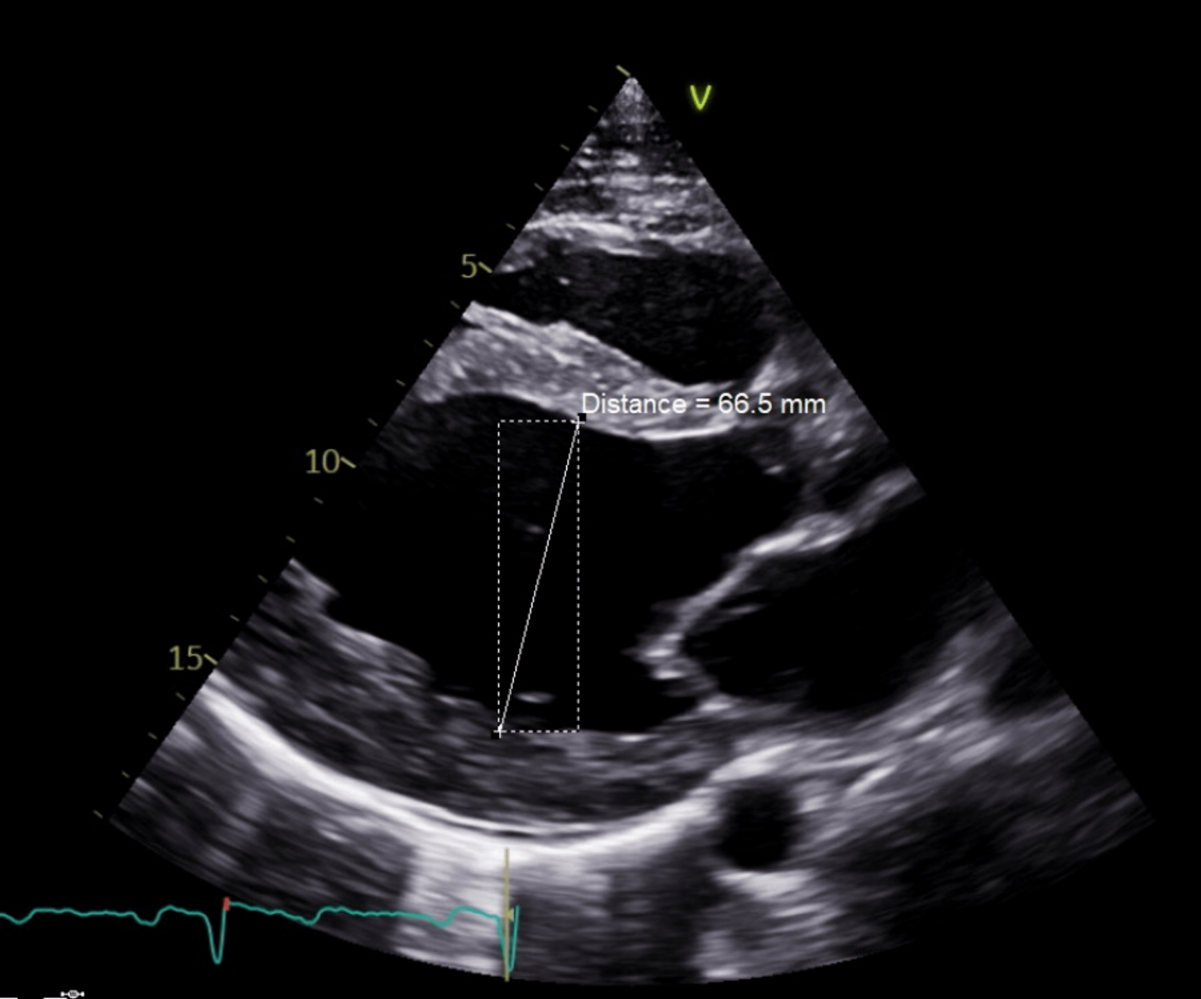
Transthoracic echocardiogram (parasagittal view) showing the left ventricular cavity measuring 66.5 mm, with an end-diastolic volume of 287 mL, suggestive of left ventricular cavity dilation.
